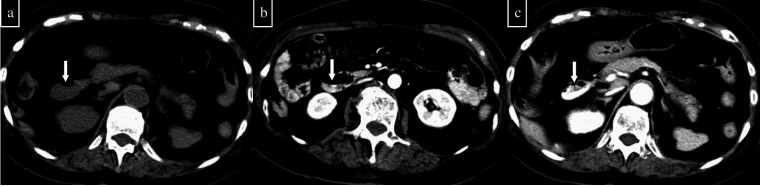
An 87-year-old woman had progressive anaemia and underwent pre-contrast and post-contrast two-phase (arterial and delayed phase) CT. (a) On the pre-contrast CT, it is difficult to determine whether the duodenal contents (arrow) are haematomas. (b) The arterial phase shows extravasation into the duodenal lumen. (c) Extravasation increases in the delayed phase. On the basis of these findings, duodenal active bleeding is suspected.
